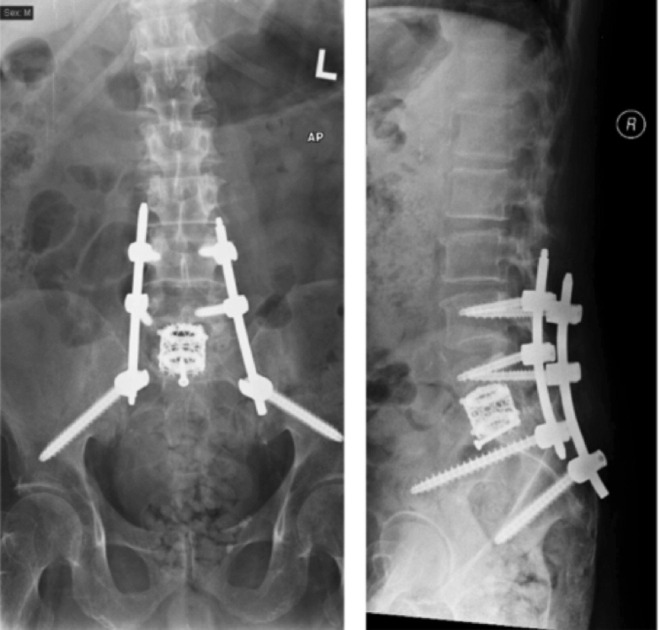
Post-operative radiographs of anteriorposterior and lateral views of lumbo-sacral spine.
